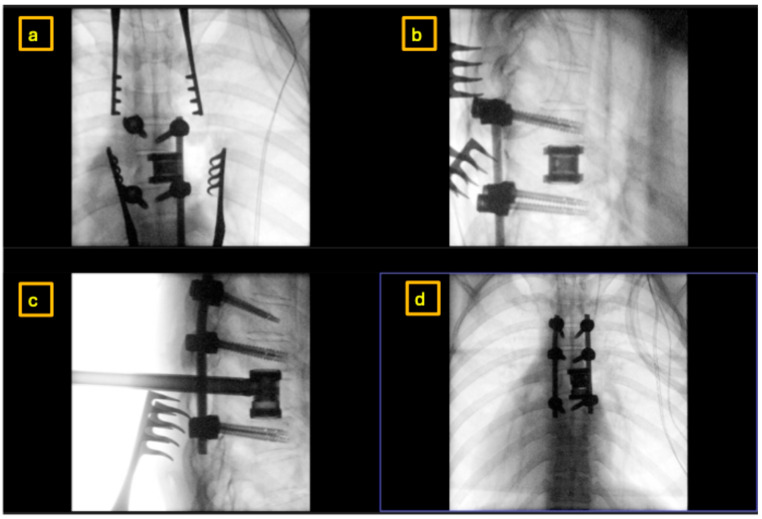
Intraoperative X-Rays showing placement of the expandable cage at the T7 level from the right via a posterolateral approach: (a,d) anteroposterior views; (b,c) lateral (sagittal) views.
